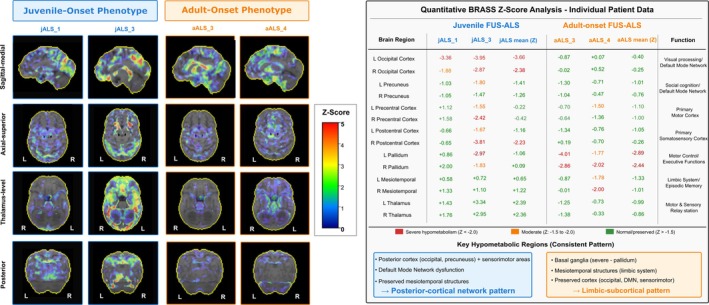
FDG‐PET reveals distinct metabolic patterns in jALS vs. aALS FUS‐ALS. (Left panel) Representative FDG‐PET z‐score maps for two juvenile‐onset patients (jALS_1, jALS_3; blue frame) and two adult‐onset patients (aALS_3, aALS_4; orange frame) displayed in sagittal‐medial, axial‐superior, thalamus‐level, and posterior views. Color scale represents regional metabolism z‐scores relative to age‐matched controls; warm colors (red‐orange) indicate hypometabolism, cool colors (blue) indicate normal or hypermetabolism. (Right panel) Quantitative BRASS (Brain Abnormality by Statistical Segmentation) z‐score analysis for individual patients across functionally relevant brain regions. Z‐scores < −2.0 (red) indicate severe hypometabolism, −2.0 to −1.5 (orange) moderate hypometabolism, and > −1.5 (green) normal/preserved metabolism. jALS patients demonstrate severe posterior‐cortical hypometabolism while aALS patients show predominantly limbic‐subcortical hypometabolism with relative preservation of cortical metabolism. Abbreviations: FDG‐PET, fluorodeoxyglucose positron emission tomography; BRASS, Brain Abnormality by Statistical Segmentation; L, left; R, right; jALS, juvenile‐onset ALS; aALS, adult‐onset ALS.
